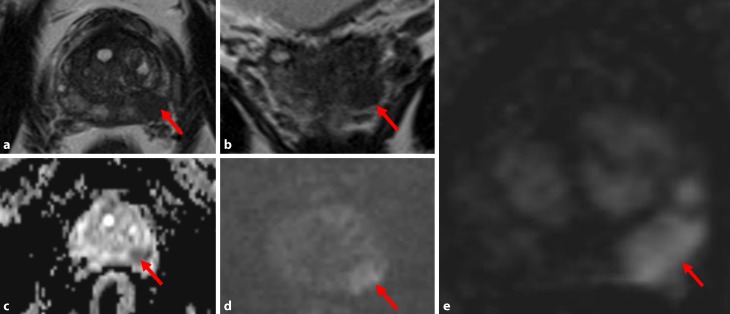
Prostate carcinoma in the peripheral zone (*red arrow*): hypointensity on axial T2-weighted (a) and on follow-up T2-weighted images (b); hypointensity on ADC (Apparent Diffusion Coefficient) maps (c) and hyperintensity on diffusion-weighted images (d); marked early contrast enhancement on dynamic contrast-enhanced sequences (e)
